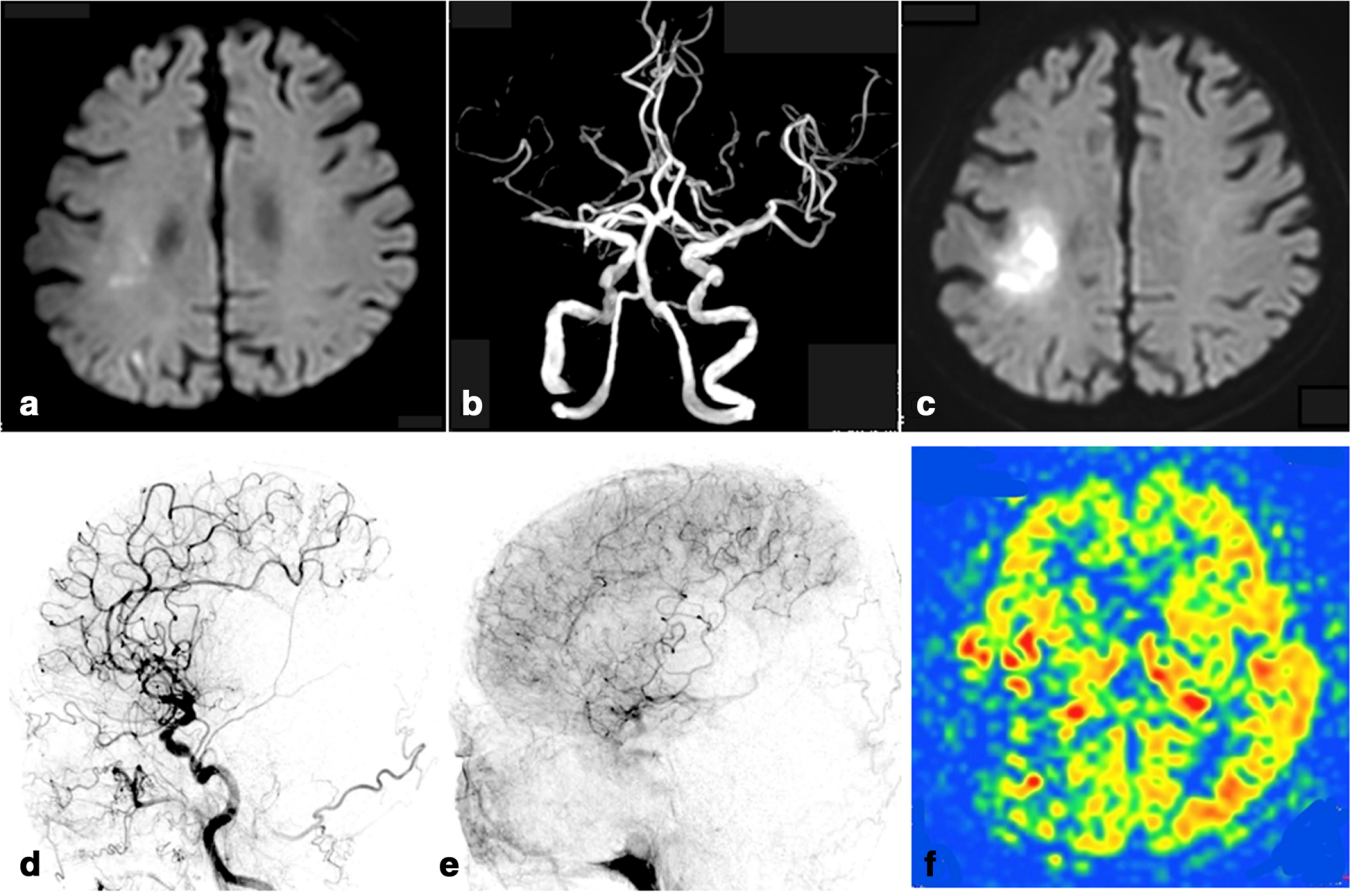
Preoperative investigations. (a) Magnetic resonance imaging (MRI) on admission shows a small infarction in the left parietal lobe on diffusion-weighted imaging (DWI). (b) MR angiography on admission shows occlusion of the M2 inferior trunk of the middle cerebral artery (MCA). (c) Serial MRI shows expansion of cerebral infarction on DWI. D, E: Lateral view of right carotid angiograms. (d) In the early phase, there is an avascular area in the territory of the M2 inferior trunk. (e) In the late phase, vessels that belong to the M2 inferior trunk are represented by retrograde flow from the anterior cerebral artery. (f) Arterial spin labeling (ASL) perfusion imaging showed decreased intensity in the M2 inferior trunk of the right MCA territory
